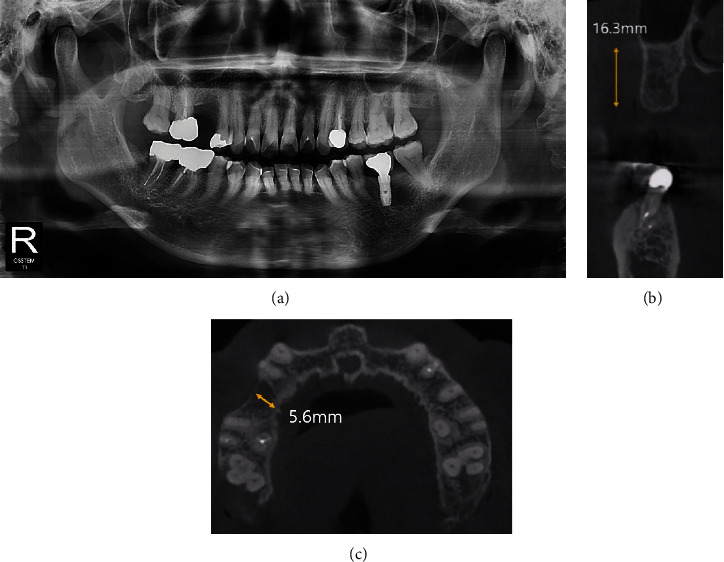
Pre-operative radiographic views (Case 1). (a) Panoramic view. (b) and (c) CBCT view. The height of alveolar bone was sufficient, but it can be seen that the width was somewhat narrowed.
